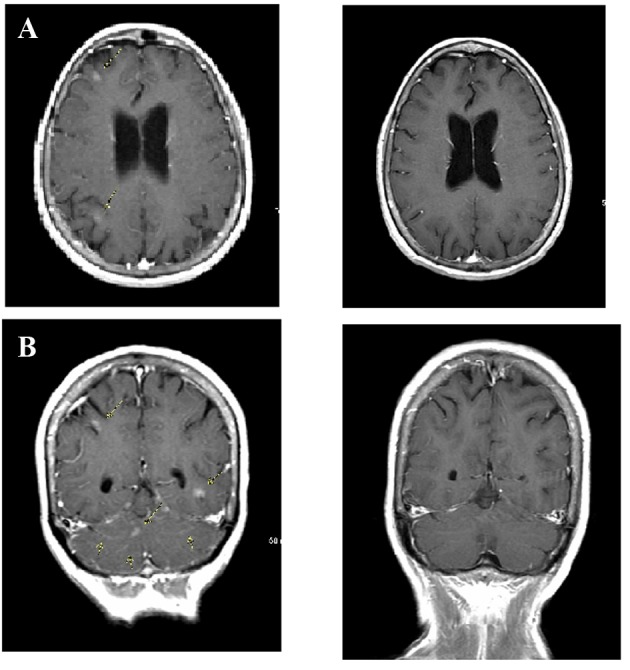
Leptomeningeal carcinomatosis. (A) Brain magnetic resonance imaging (MRI) obtained when patient first presented with neurologic deficits shows multiple sites of leptomeningeal enhancement on post-contrast T1-weighted sequences (arrows). (B) Following pulsed-dose erlotinib therapy, repeat MRI scan showed complete resolution of tumor.
